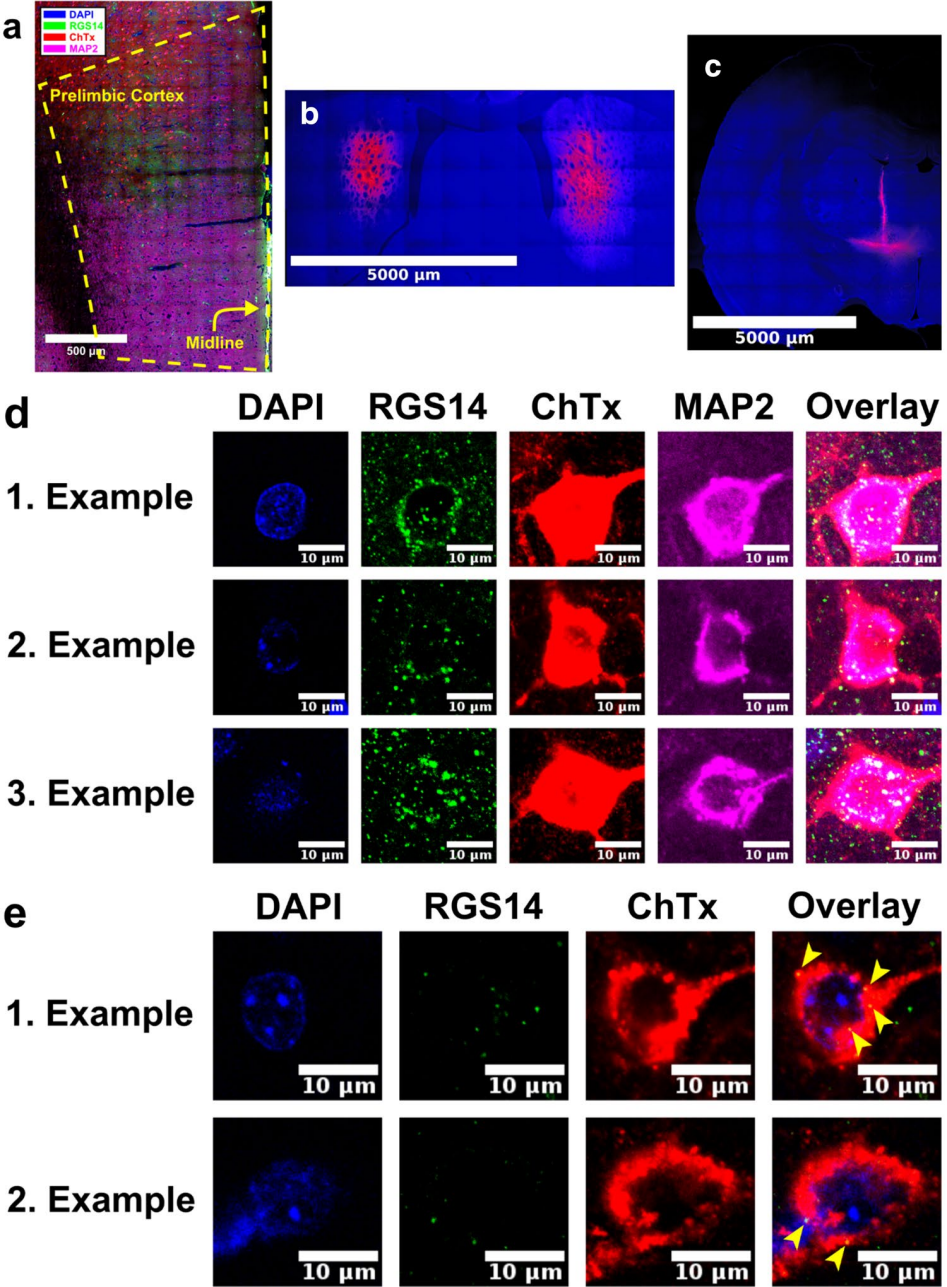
RGS14 expression in prelimbic cortex. a CS neurons that were retrogradely labeled with choleratoxin subunit B (ChTx, red) express RGS14 (green) and MAP2 (magenta) in prelimbic cortex, which is outlined by the yellow dashed line. b Injection site of choleratoxin subunit B (red) in dorsomedial striatum. c Injection site of choleratoxin subunit B (red) in ventral motor and adjacent thalamic nuclei. d CS neurons in prelimbic cortex that were retrogradely labeled with choleratoxin subunit B (ChTx; red) from dorsomedial striatum express RGS14 (green) and MAP2 (magenta), while e CT neurons do not express RGS14 (green) in the somata. Yellow arrowheads mark sparse expression of RGS14 along the outer membrane of the cell nuclei or in the somata that was observed in very few CT neurons in prelimbic cortex. Cell nuclei are counterstained with DAPI (blue)
